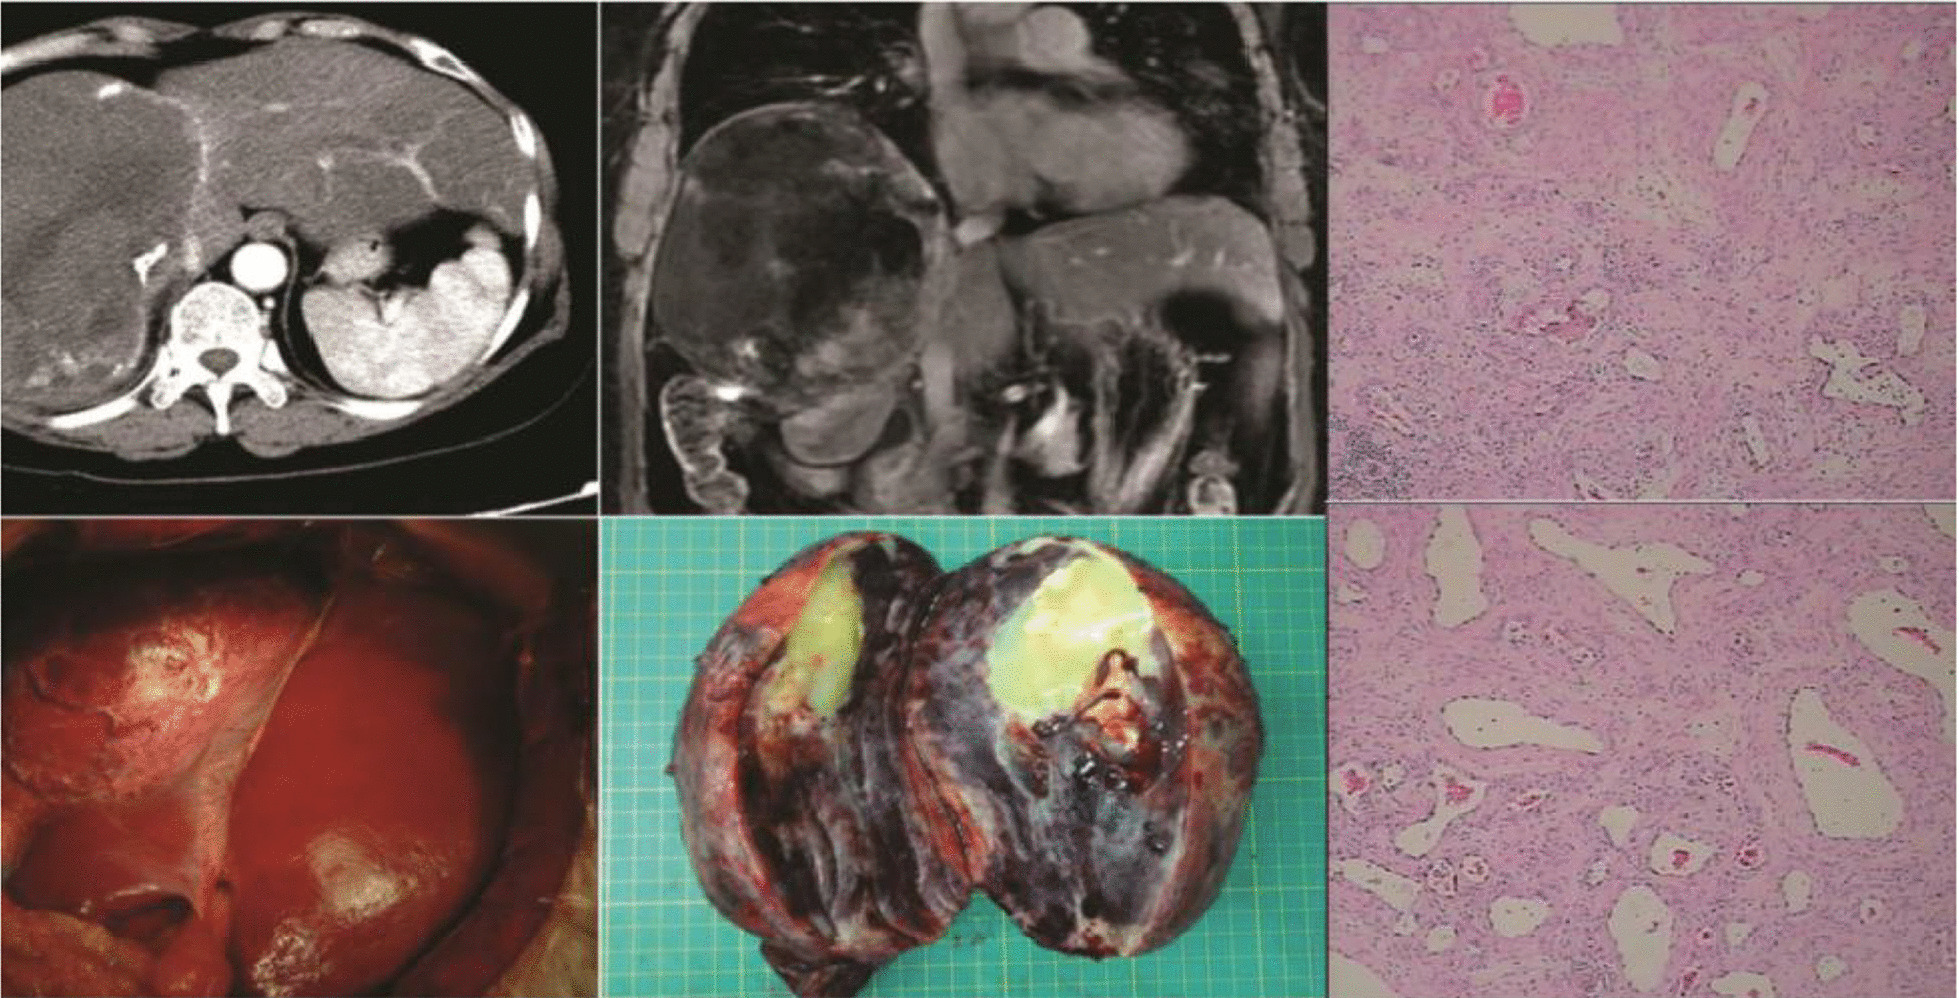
A 62-year-old female patient with a giant hemangioma in the right lobe of the liver was misdiagnosed with hepatocellular carcinoma. In 2006, the patient underwent physical examination, which revealed a liver mass. The patient was diagnosed with hepatocellular carcinoma and underwent five rounds of transhepatic arterial chemotherapy and embolization and multiple cytokine-induced killer cell treatments at another hospital. In December 2015, the patient was admitted to our hospital and we determined that she was not infected with hepatitis b virus and was α-fetoprotein negative. The CT image shows a large mass in the right lobe of the liver. The patient’s liver reserve function was normal. She underwent extensive right hepatectomy and was given a final pathological diagnosis of hepatic hemangioma
